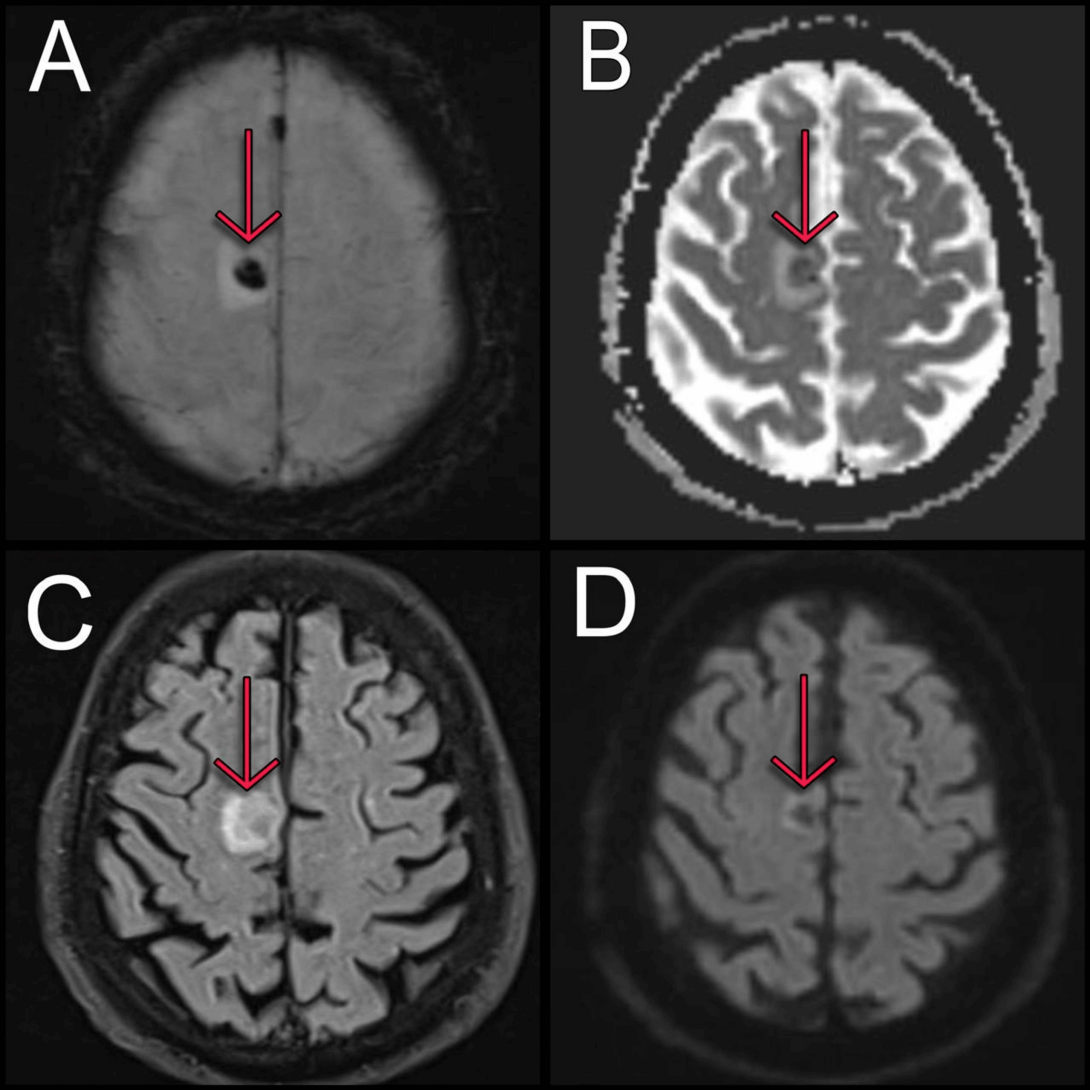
Magnetic resonance imaging sequences of the brain (A) Susceptibility-weighted imaging (SWI) of the brain showing right superior frontal gyrus involvement. (B) Apparent diffusion coefficient (ADC) showing a hypointense lesion in the right superior frontal gyrus. (C) Fluid-attenuated inversion recovery (FLAIR) reported a hyperintense lesion in the right superior frontal gyrus. (D) Diffusion-weighted imaging (DWI) revealing a hypointense lesion in the right superior frontal gyrus.
